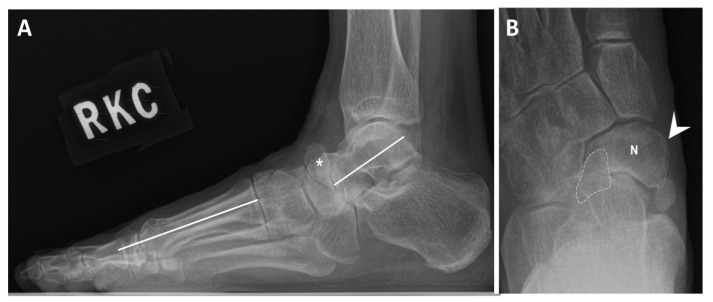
The measurement of Meary–Tomeno (M–T) angle at the lateral standing foot radiograph (solid white lines) (A) and radiographic features on DP view (B) of MWS foot in 49-year-old female. The measured M–T angle was 10.1 degree. This case was classified as Maceira stage 4 (asterisk: splitting of navicular; arrowhead: medial protrusion of navicular; N: navicular; dotted line: fragmentation of lateral navicular).
